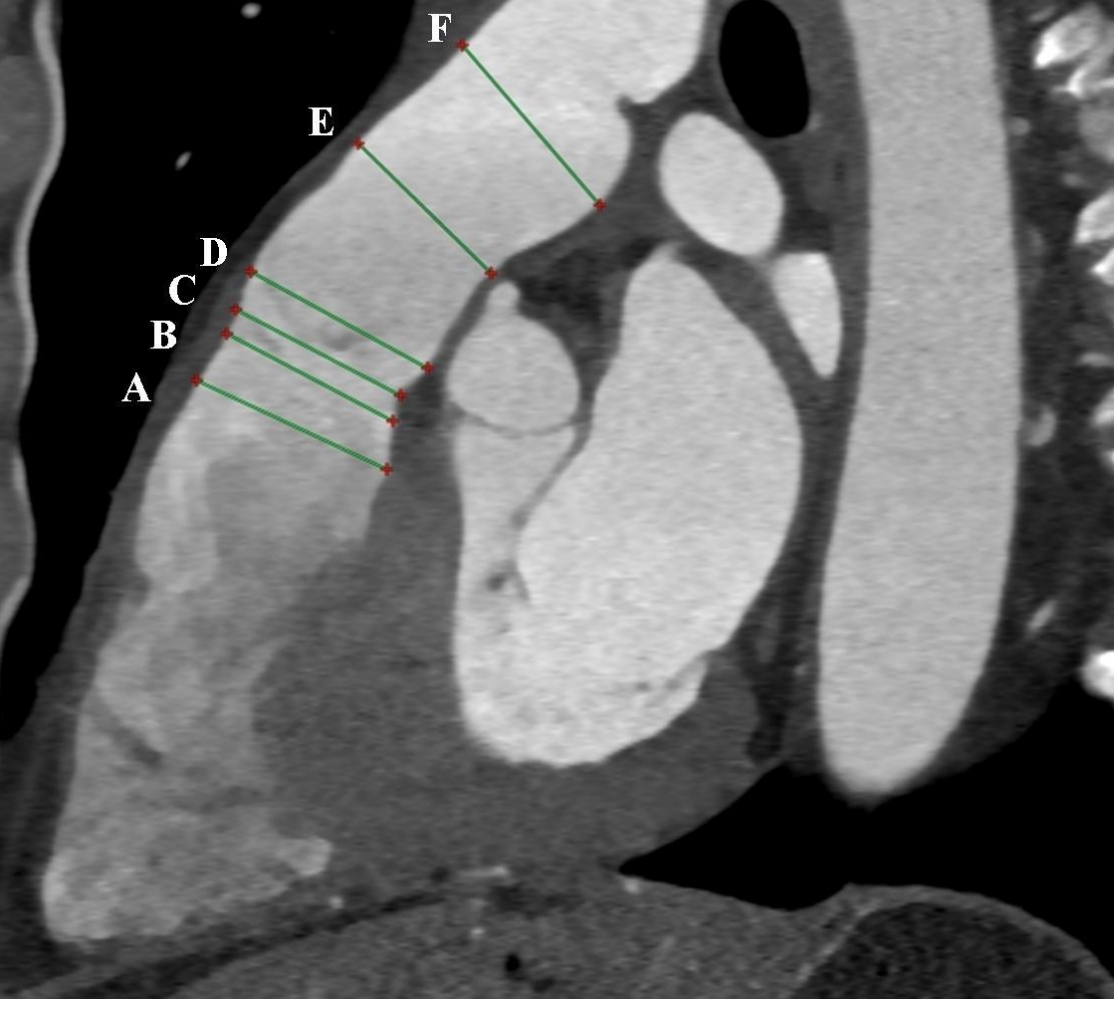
Cardiac computed tomography before transcatheter pulmonary valve implantation. MPR reconstruction. The green lines indicate 6 consecutive dimensions levels: A—level of the right ventricular outflow tract; B—level under the pulmonary valve; C—level of the pulmonary valve; D—level above the pulmonary valve; E—level of the middle part of the main pulmonary artery; F—level before the bifurcation main pulmonary artery.
